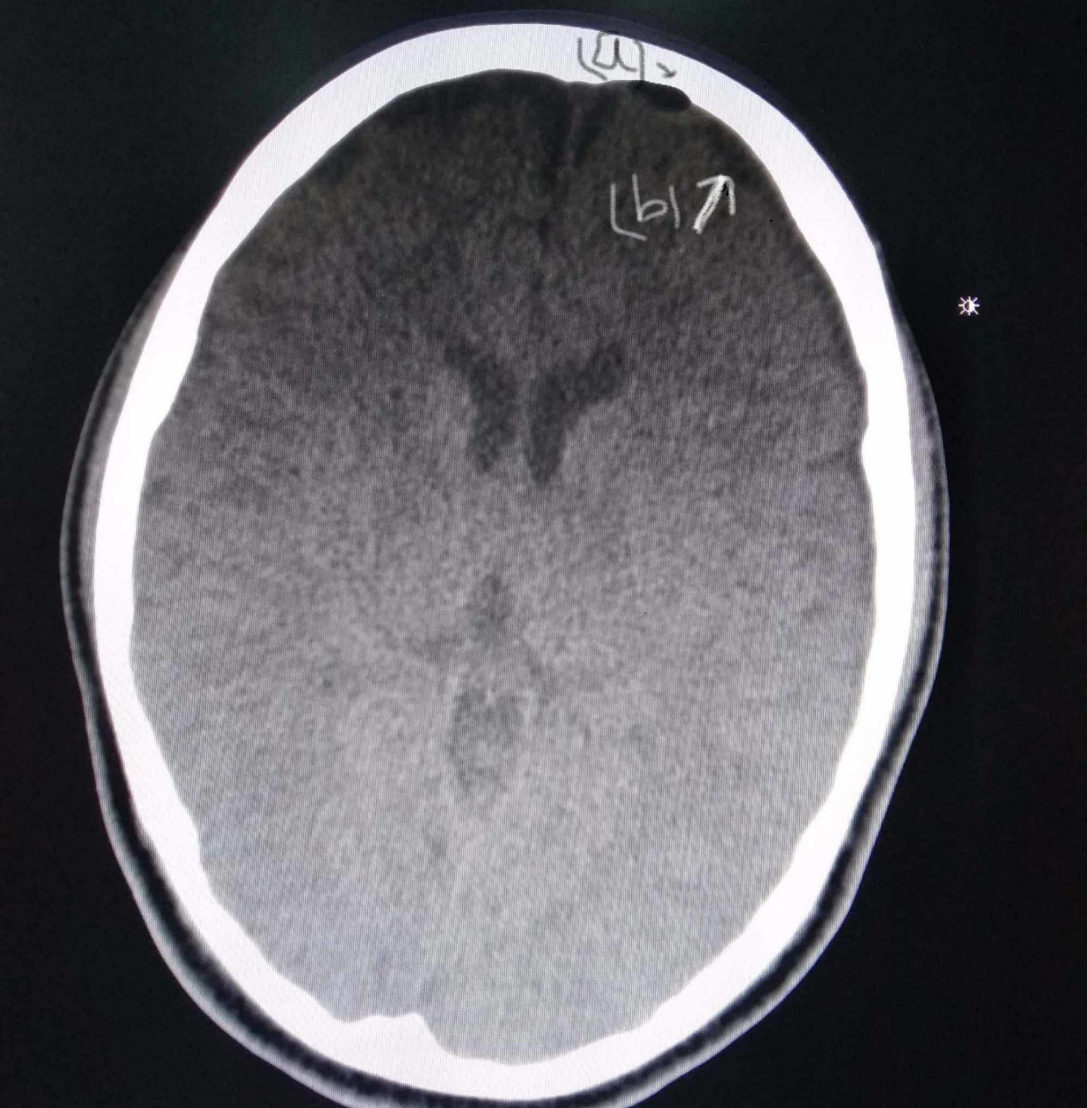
Axial CT scan; (a) pneumocephalus and (b) subdural empyema
